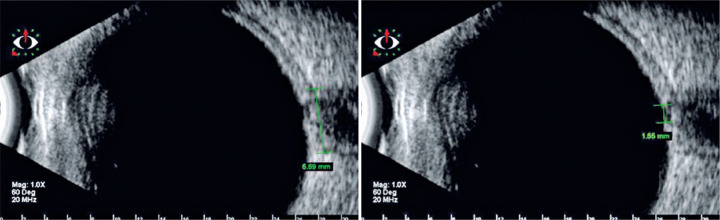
Ultrasonography image of the optic nerve head.
